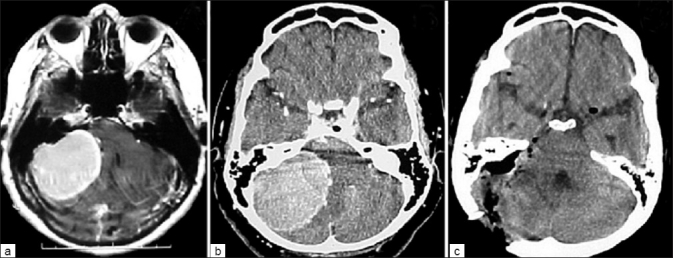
Tumor located at 6B removed by retrosigmoid approach; (a) preoperative MRI (b) preoperative CT scan (c) postoperative CT scan showing total macroscopic removal of the meningioma
